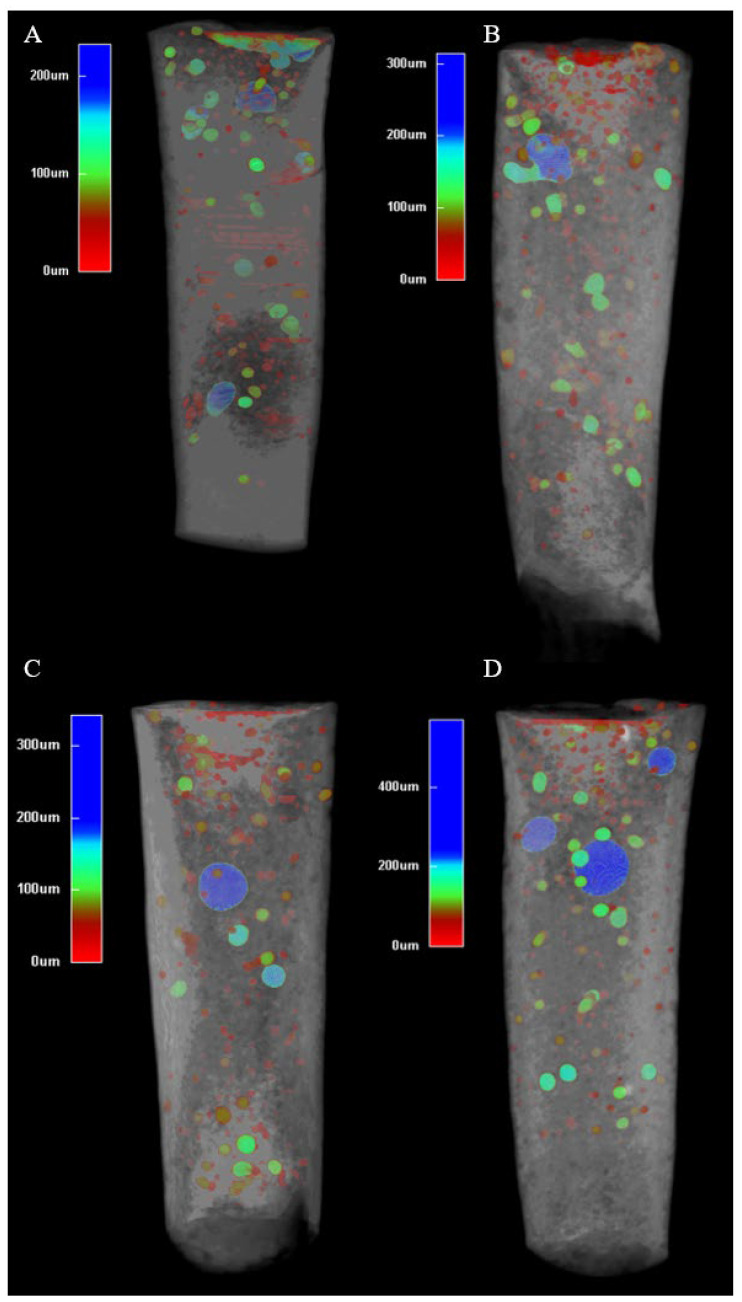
Representative micro-CT images of apical plugs, created with different condensation techniques with superimposed voids (color-coded depending on their size). (A) Manual technique; (B) ultrasound condensation for 3 s; (C) ultrasound condensation for 9 s; (D) ultrasound condensation for 15 s.
